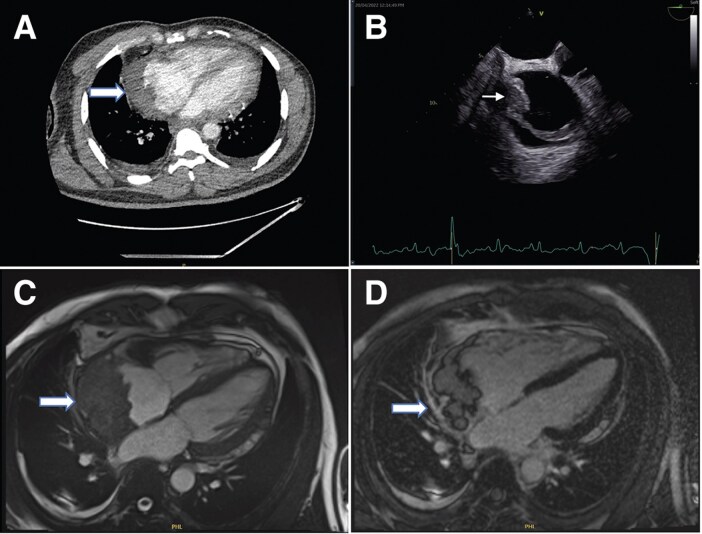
(*A*) Contrast computed tomography thorax (using Omnipaque 350 contrast agent) showing a filling defect in the right atrium’s lateral wall. (*B*) This was also seen in the transoesophageal echo as seen in the image on the right. (*C*) Four-chamber balanced SFPP Cine sequence revealed a large mass (6 × 3 cm) with irregular margins which appears to originate from the pericardium and compressing the right atrium. (*D*) Four-chamber delayed gadolinium enhancement sequence showed heterogeneous enhancement of the mass and no myocardial late gadolinium enhancement.
